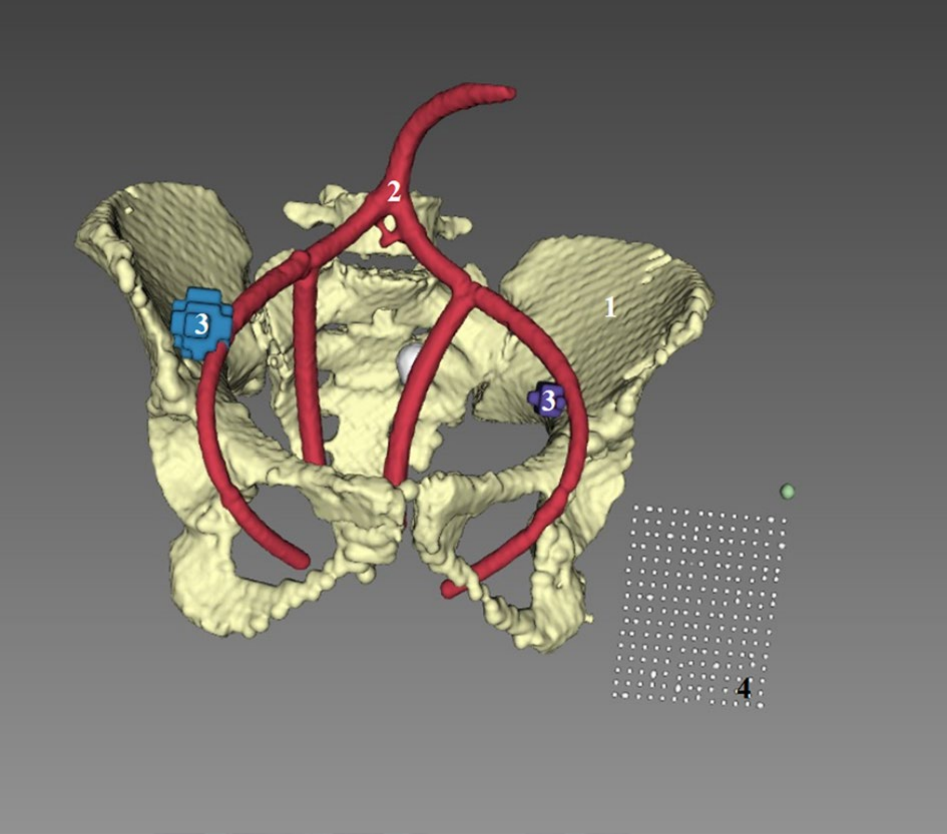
Results of the segmentation process, after registration. Segmentation of CT images with 3D reconstruction of pelvic structures (pelvic bones (1), arteries (2), lymph nodes (3), and calibration device (4). CT, computed tomography
